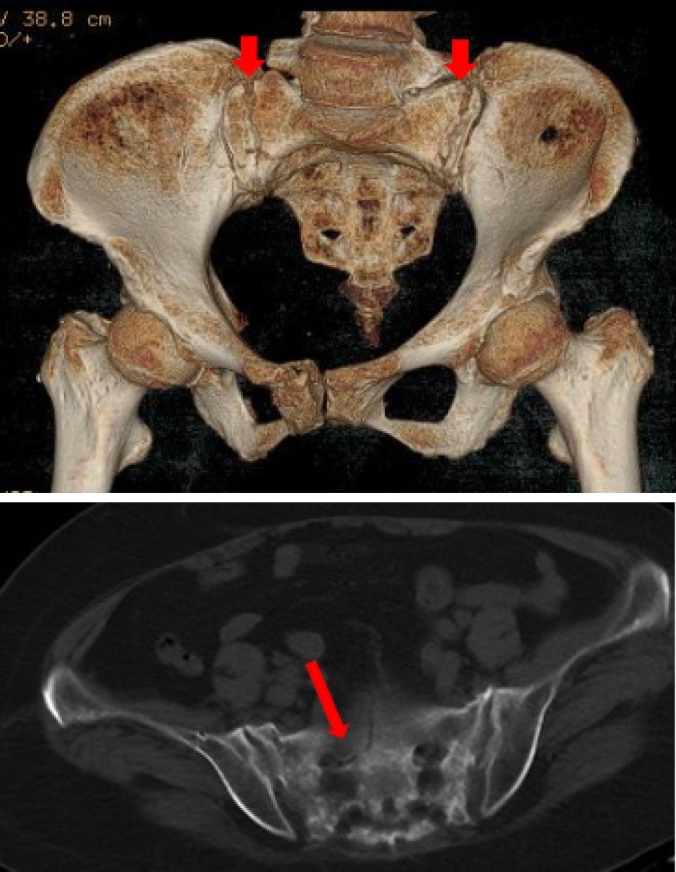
CT examination showed sacrum fracture in both alae of sacrum and vertebral body of S1. CT, computed tomography.
